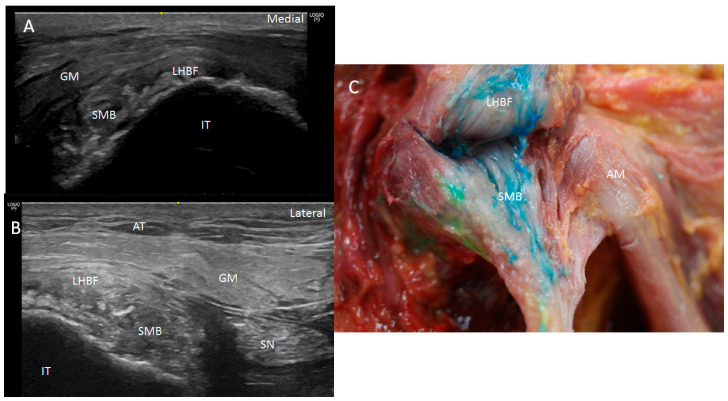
(A,B) Two ultrasound examples in the short axis of the tendons of the long head of biceps femoris (LHBF) and the semimembranosus (SMB) muscles, presenting an irregular origin at the ischial tuberosity (IT) with several hyperechogenic structures that were compatible with a degeneration of the tendon: (A) left side; (B) right side. (C) Dissection of the left area showing, after moving the LHBF, a very irregular insertion of the tendon of the SMB with all the blue dye widespread. AM: adductor maximus muscle.
